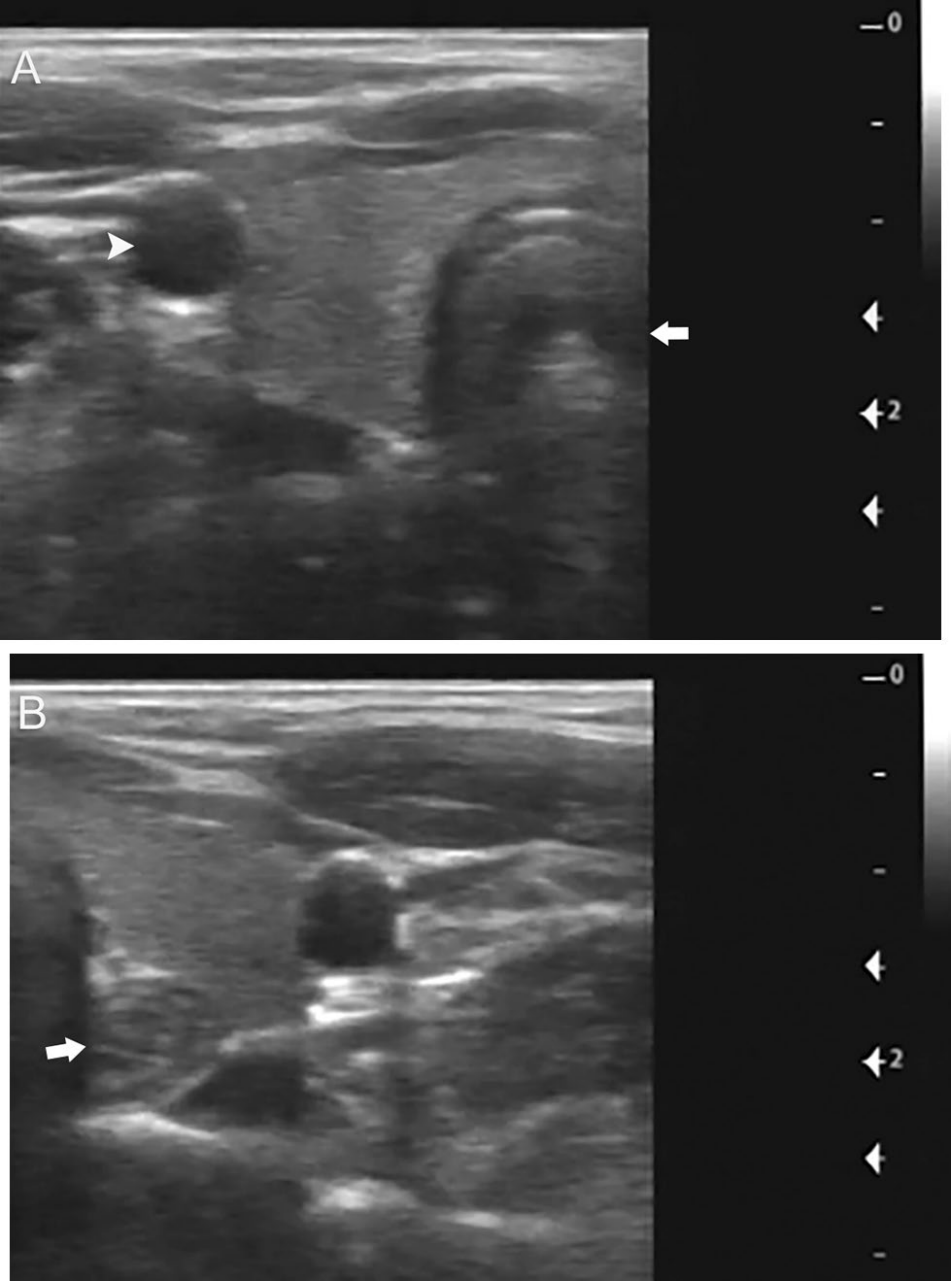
Normal thyroid on VSI. Thyroid VSI examination from an asymptomatic 65-year-old female performed by an operator after 8 h of training. a Normal right thyroid gland on a still image from the right transverse VSI cine clip. The trachea (arrow) and carotid artery (arrowhead) are also seen. b Normal left thyroid gland on a still image from the left transverse VSI cine clip. The esophagus is also seen (arrow). Supplemental Material 3 includes the cine clips from this exam. These cine clips and all other VSI examinations in this study were obtained by individuals without prior ultrasound experience
